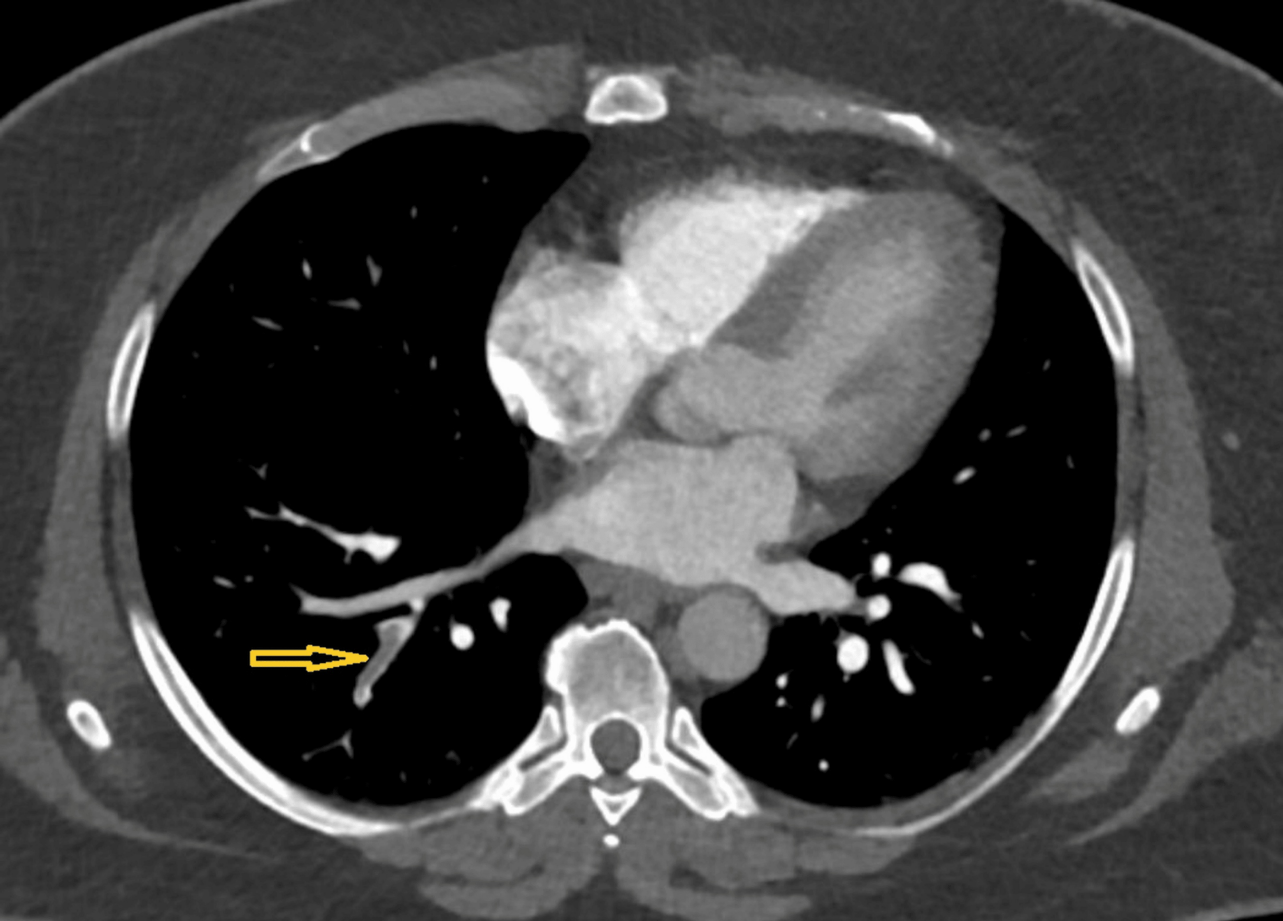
Contrast-enhanced computed tomography (CT) pulmonary angiography demonstrating acute pulmonary embolism. Axial CT pulmonary angiography image showing a nonocclusive filling defect within a segmental branch of the right lower lobe pulmonary artery (arrow), consistent with acute pulmonary embolism. No evidence of right heart strain is observed.
